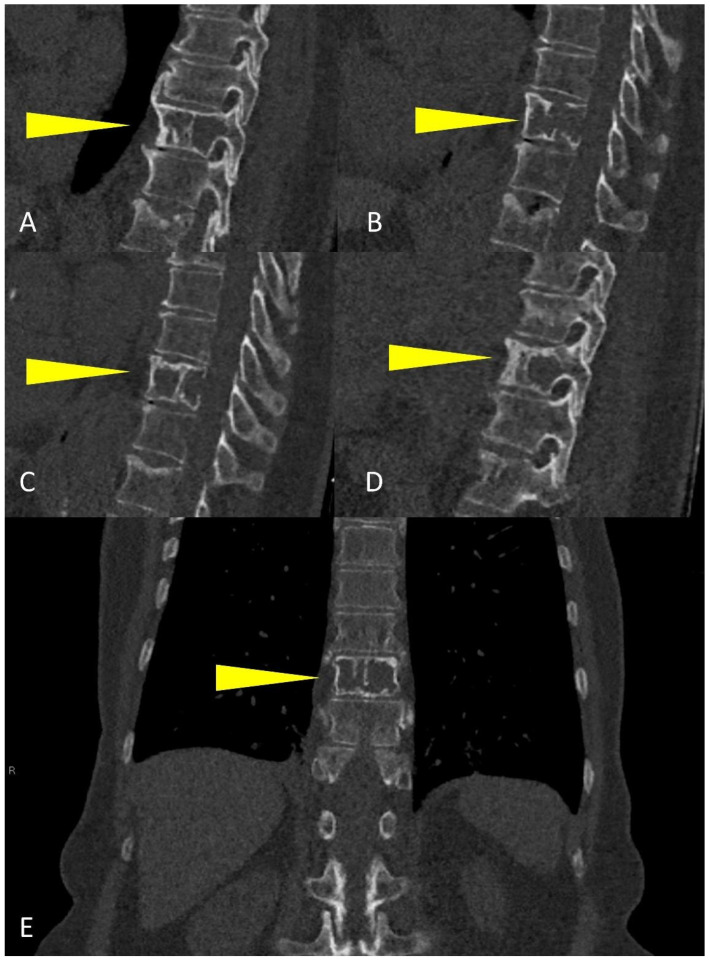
Sagittal CT reconstruction from right to left (A–D), and (E) coronal multiplanar reconstruction of a dorsal vertebra showing a large osteolytic lesion involving more than 50% of the vertebral body (yellow arrowheads), with high risk of fracture.
